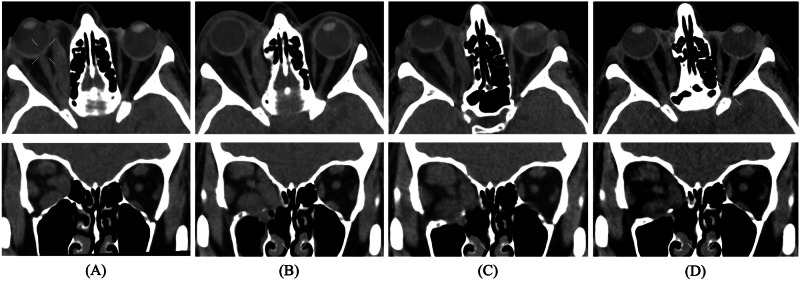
Orbital CT scans of the patient before and after treatment. Orbital CT scan (soft tissues) in axial and coronal sections before (A) and after (B) surgical decompression and before (C) and after (D) OR
